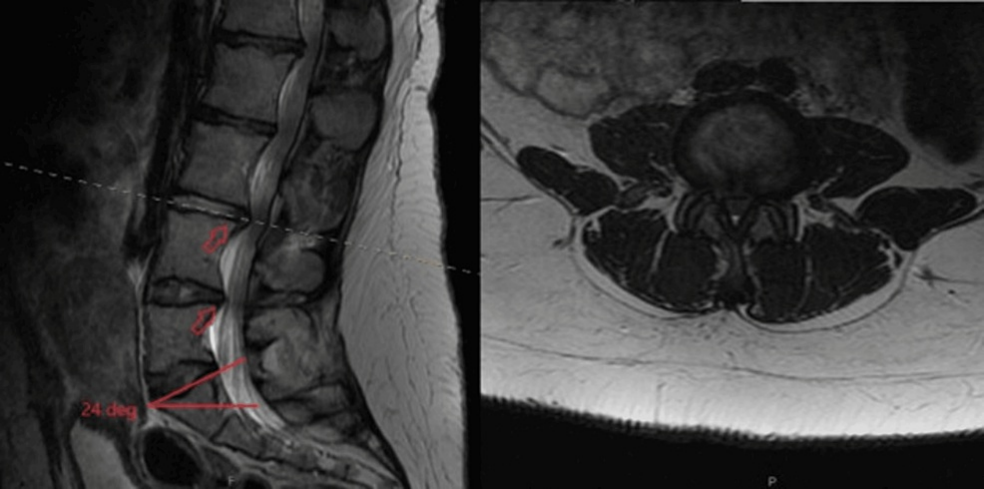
Preoperative T2-weighted Lumbar MRI. Left image (sagittal view): Broad disc bulges (red arrows) at L3/L4 and resulting descending lumbar nerve root compression; Ferguson angle is 24 degrees. Right image (axial view of L3/L4): severe canal stenosis and facet arthropathy with advanced lateral recess stenosis can be seen.
